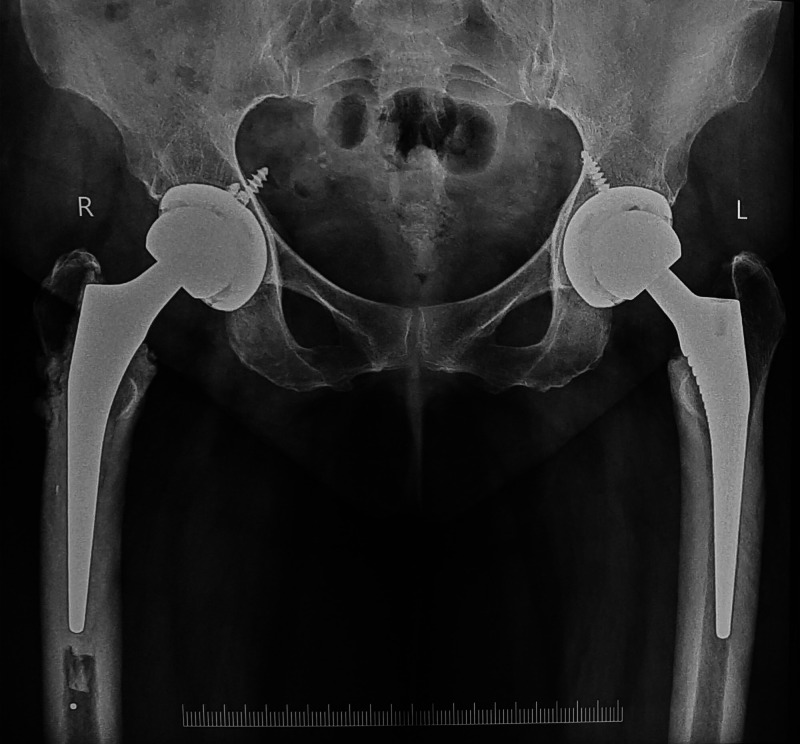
Anteroposterior both hips radiograph at two years follow up with bilateral total hip arthroplasty components in good alignment and no loosening
